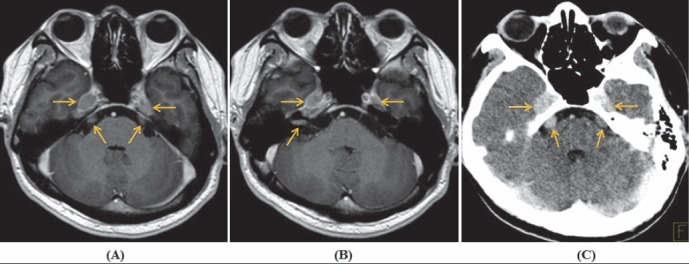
Axial contrast-enhanced T1W MRI (A, B) and CT image (C) showed enlargement and enhancement ofbilateral cranial nerve V and Gasser’s ganglions. Complex of the right cranial nerve VII/VIII was seen only on T1W image (B)
